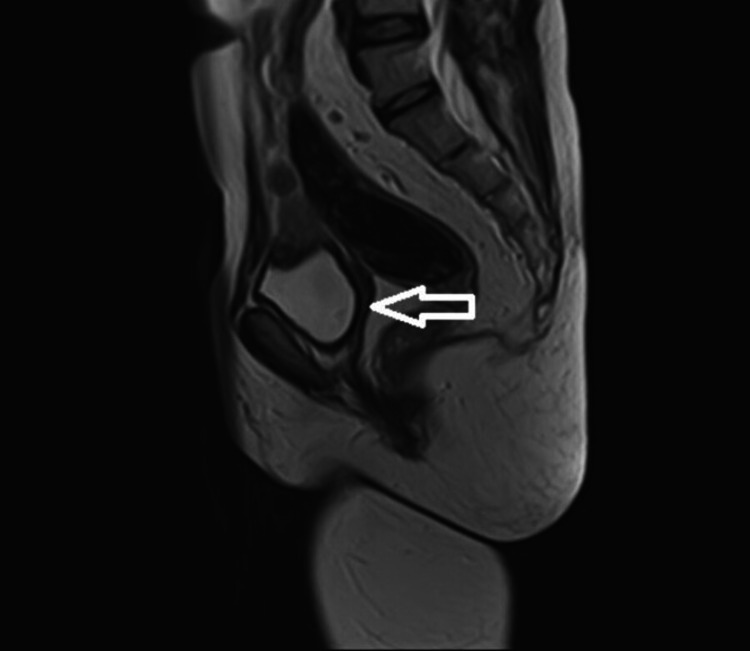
MRI abdomen and pelvis (arrow showing rudimentary uterus) MRI: magnetic resonance imaging
